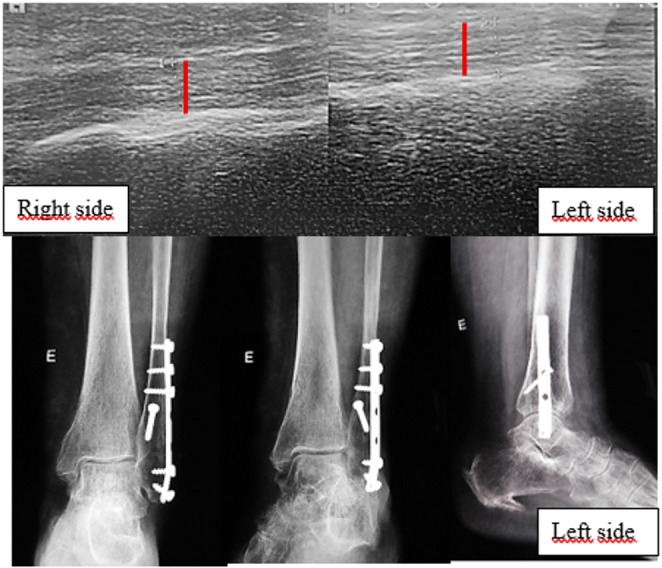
Ultrasound and plain radiographs of a left ankle fracture comparing the injured and contralateral tendons
